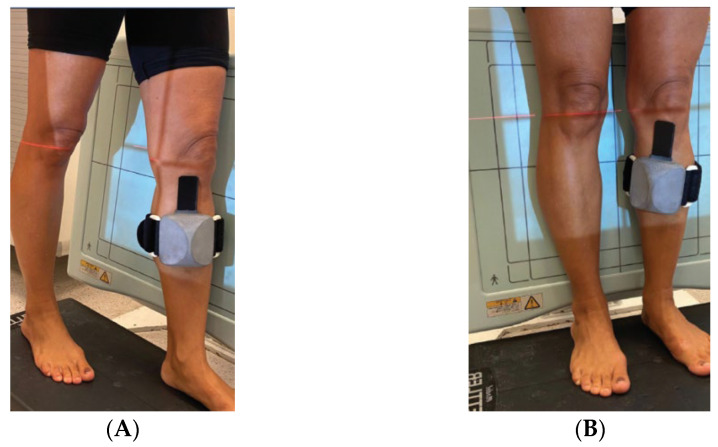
RSIP XPlan.ai™ calibration jig positioning. (A) Patient’s pose for lateral X-ray. (B) Patient’s pose for anteroposterior X-ray.
